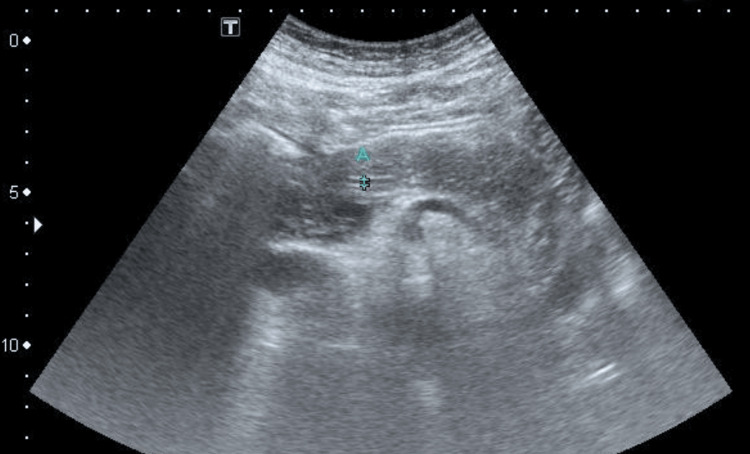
Abdominal ultrasonography demonstrating mildly heterogeneous pancreatic parenchyma and a prominent main pancreatic duct. Calipers at point A indicate measurement of the ductal diameter, approximately 2.3 mm.
